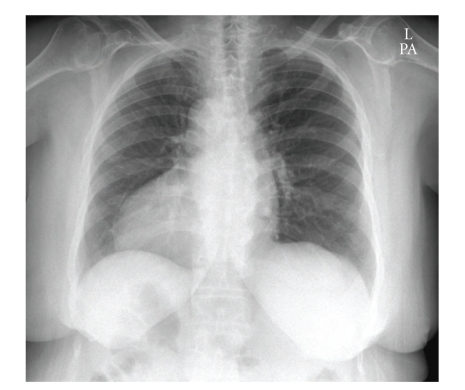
Chest radiograph confirming dextrocardia with right-sided aortic arch and right-sided gastric bubble.
